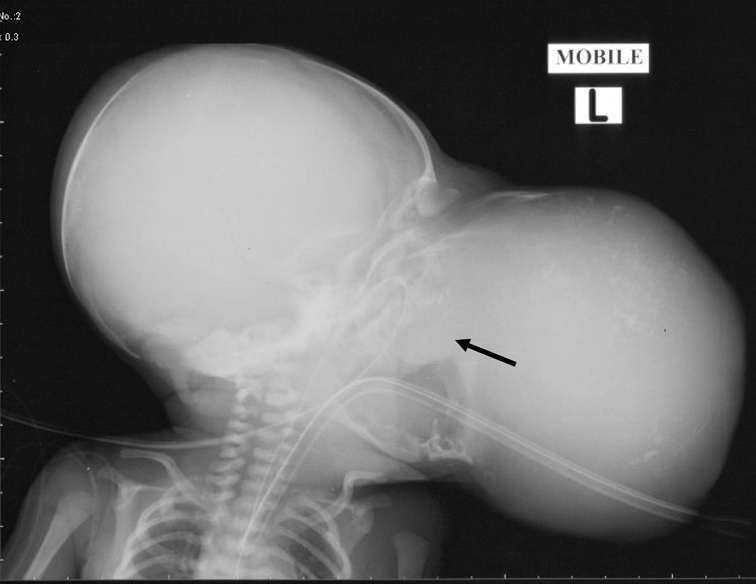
Plain radiograph of the head showing a large soft tissue mass protruding from the anterior aspect of the face with calcifications within it. Note the under-developed left temporomandibular joint (black arrow).
